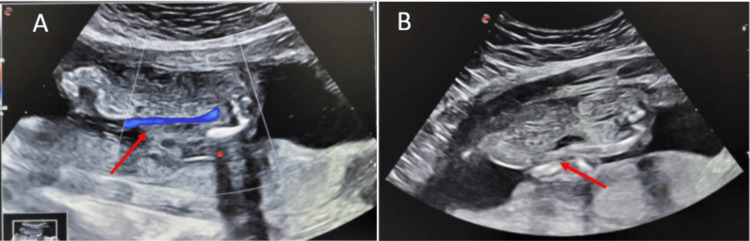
Prenatal ultrasound demonstrating omphalocele and umbilical cord abnormality. (A, B) B-mode antenatal ultrasound at the level of the fetal abdomen showing a supraumbilical anterior abdominal wall defect with a large omphalocele (red arrows). Color Doppler demonstrates a single umbilical artery (blue signal).
